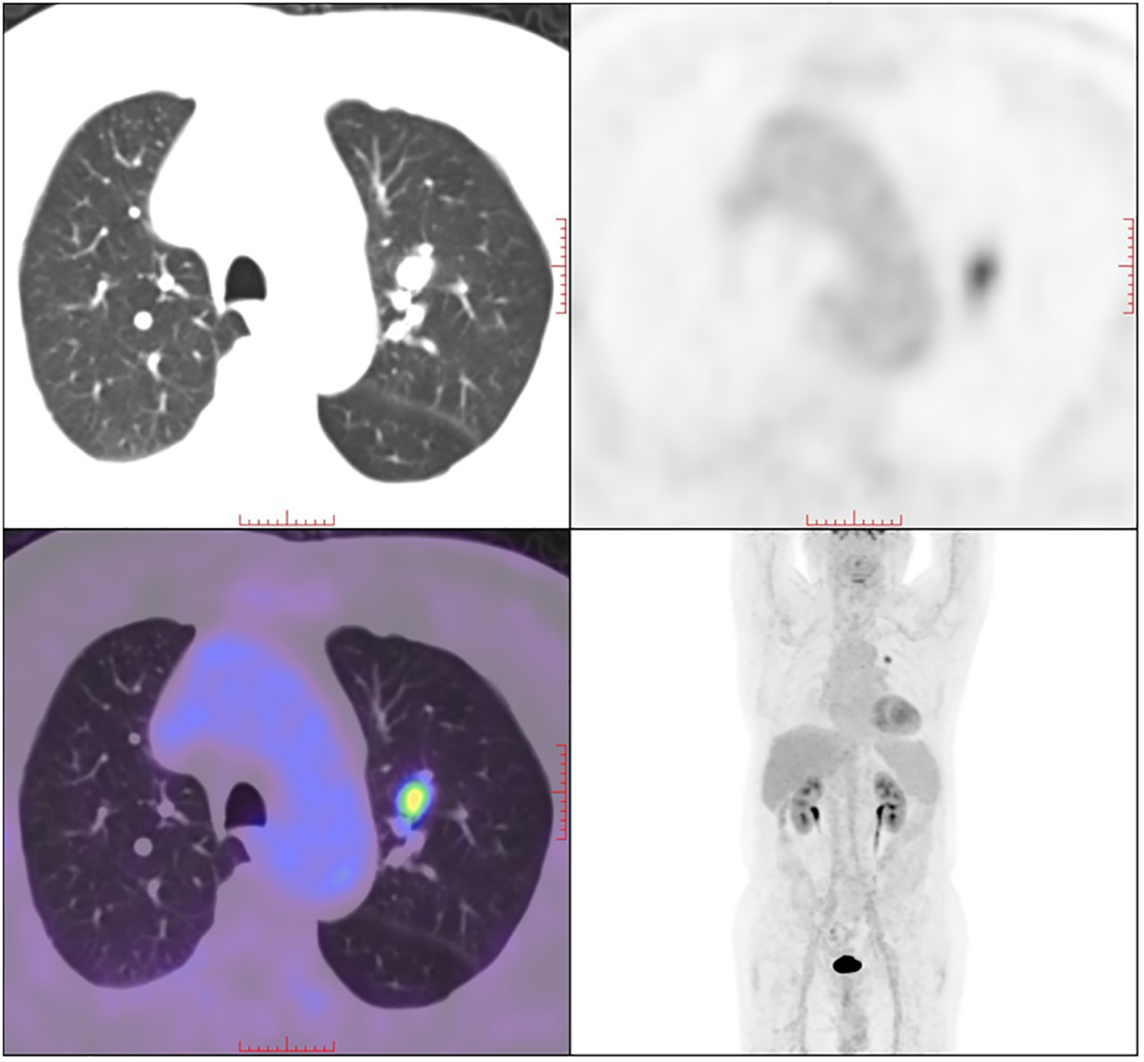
18F-FDG PET/CT scan of the patient. A solid nodule in the left upper lobe with increased 18F-FDG uptake was detected.
